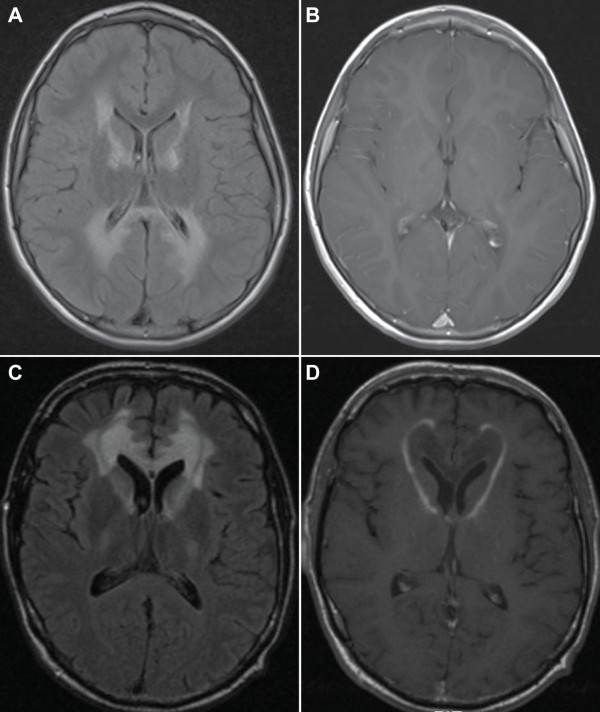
MRI of the brain in a case of childhood cerebral ALD showing characteristic extensive white matter changes in the parieto-occipital region and internal capsules on FLAIR sequences (A). This area is initially affected in about 80% of cases of cerebral ALD. The rim enhances after administration of gadolinium on T1 sequences (B). In about 20% of cases the site of initial involvement in cerebral ALD is the frontal white matter as shown on this FLAIR image of a different patient with cerebral ALD (C), with prominent rim enhancement after administration of gadolinium on a T1 weighted image (D).
